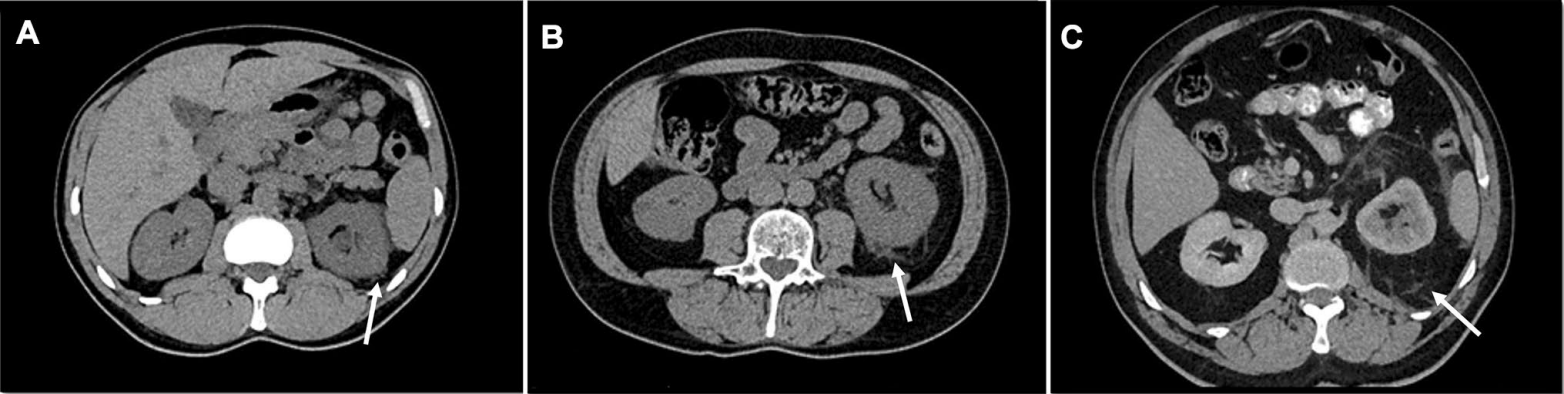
Examples of computed tomography scans with A mild, B moderate, and C severe perirenal stranding indicated by the white arrow
